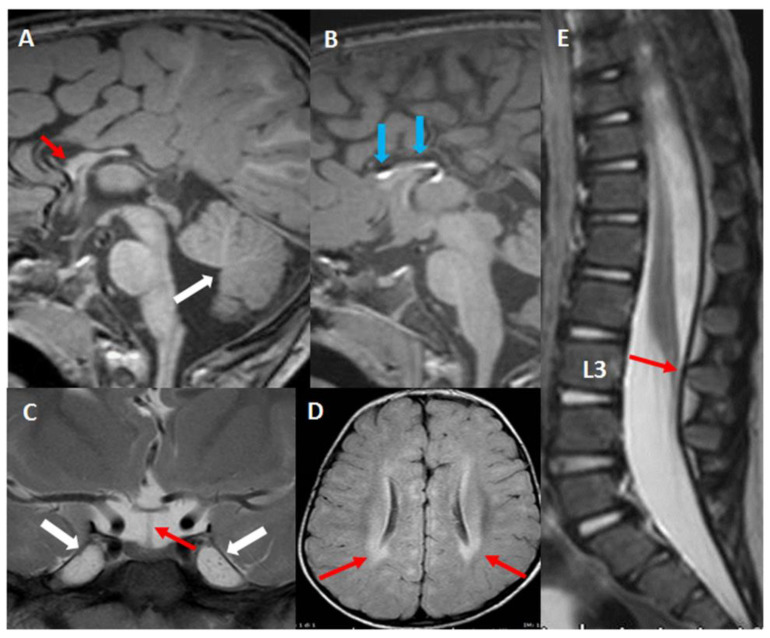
Neuroradiological findings of P149 (A,B) sagittal T1 3D TFE images showing corpus callosum partial agenesis (red arrow) and associated lipoma (blue arrows), mild cerebellar vermis extra rotation (white arrow). (C) T2 weighted coronal image Meckel’s cave ectasia (white arrows) and elongated pituitary stalk (red arrow). (D) FLAIR 2D image showing shaded and diffuse posterior white matter hyper-intensity (red arrows). (E) T2 weighted sagittal image showing low-lying conus medullaris ending at L3 (red arrow).
